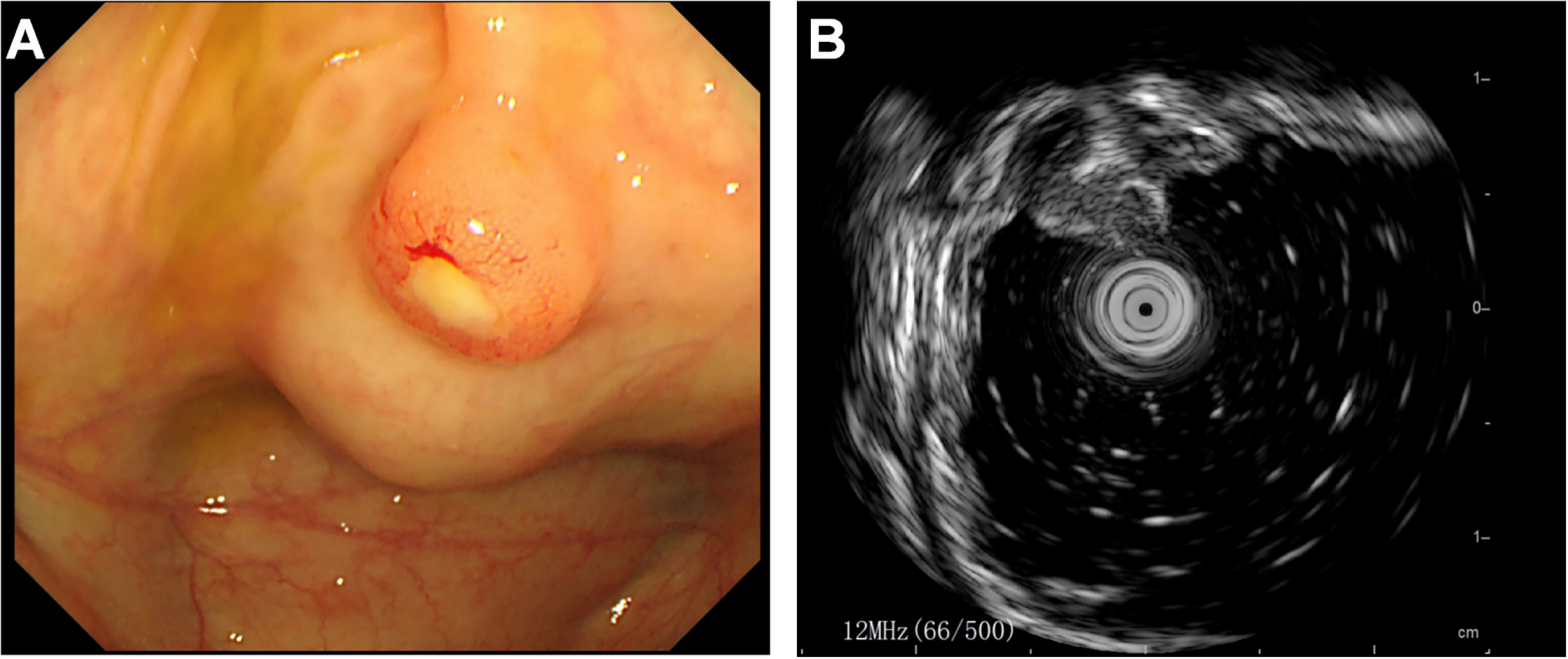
Endoscopic evaluation of appendiceal neuroendocrine tumor. (A) Colonoscopic visualization of a hemispherical protrusion at the appendiceal orifice. (B) Endoscopic ultrasound revealing a heterogeneous predominantly hypoechoic lesion (calipers) originating from the submucosal layer.
